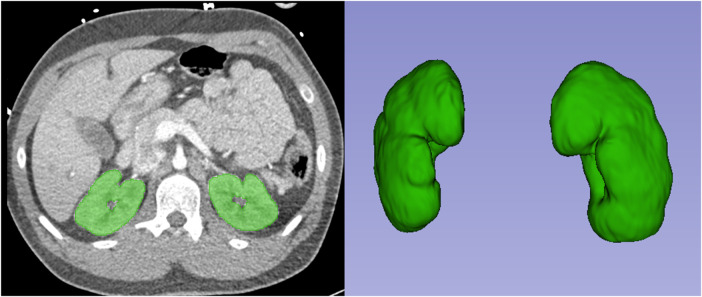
Example of kidney segmentation and 3D rendering. Axial contrast-enhanced CT slice at admission with bilateral kidney masks and 3D surface rendering of both kidneys derived from the same segmentation.
